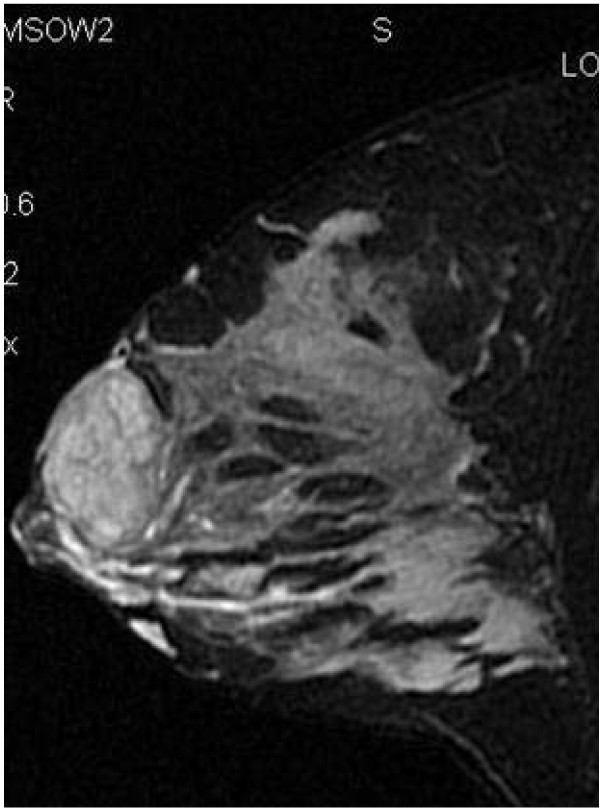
MRI: STIR sequences with fat suppression in sagittal plane.
